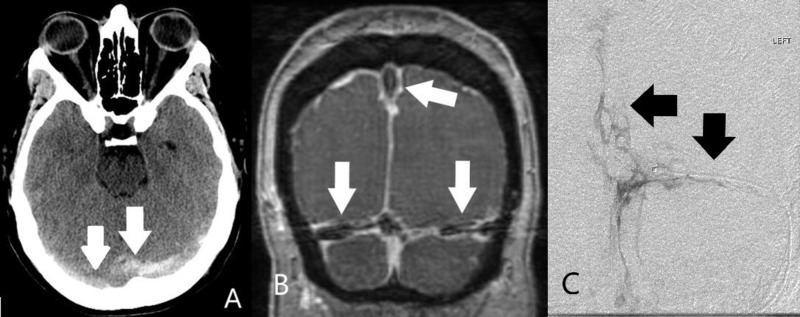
Diagnostic confirmation of multiple dural sinus thromboses with several imaging modalities. A) Noncontrast head computed tomography scan demonstrating hyperdense appearance of the bilateral transverse sinuses (arrows). B) Coronal post-contrast magnetic resonance venography at the torcula demonstrating extensive filling defects throughout the dural sinuses (arrows). C) Digital subtraction angiography image from catheter venography demonstrating extensive filling defects through the dural sinuses (arrows).
